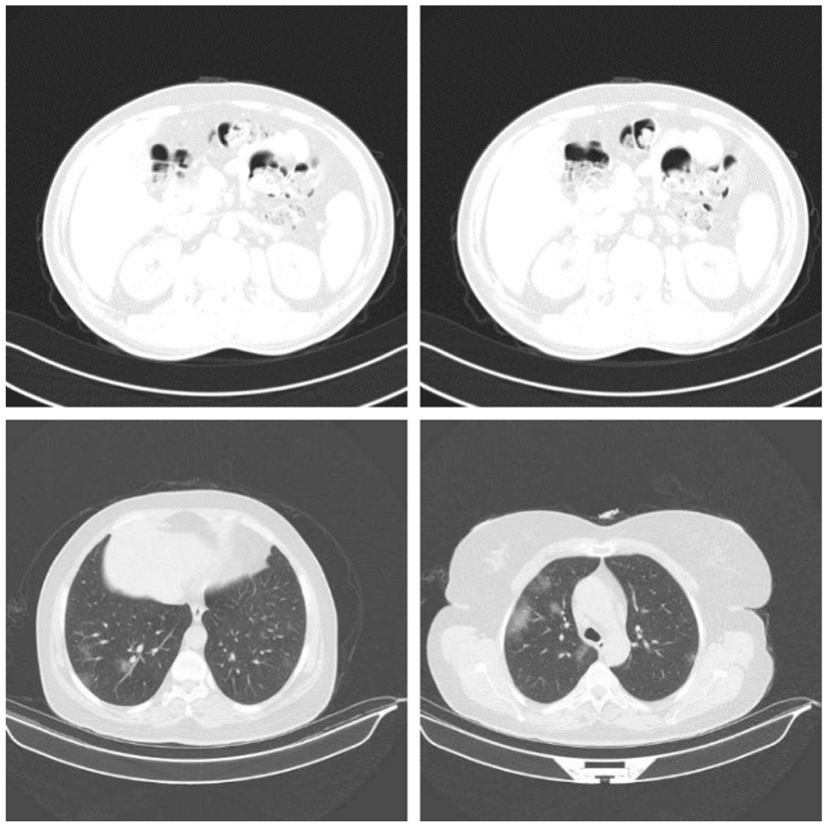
Samples of normal CT images (row 1) and COVID-19 CT images (row 2) used for evaluation (Gunraj et al., 2021).
